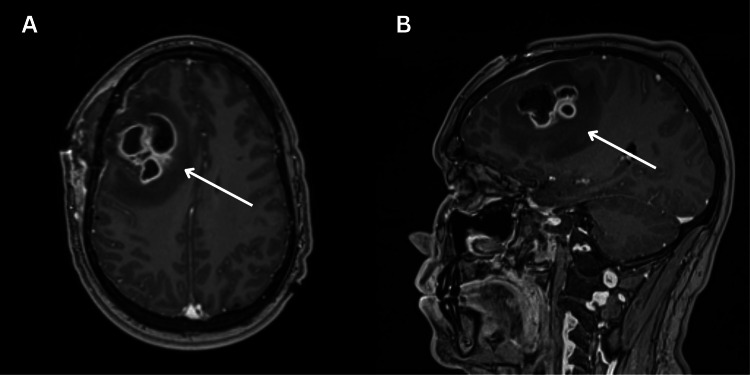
Postoperative head imaging Axial (A) and sagittal (B) view of a post-gadolinium T1-weighted brain MRI on postoperative day 4 showing a multi-loculated, rim-enhancing lesion (3.8 x 1.6 cm) in the right frontal lobe with regional mass effect and 6-7 mm leftward midline shift.
